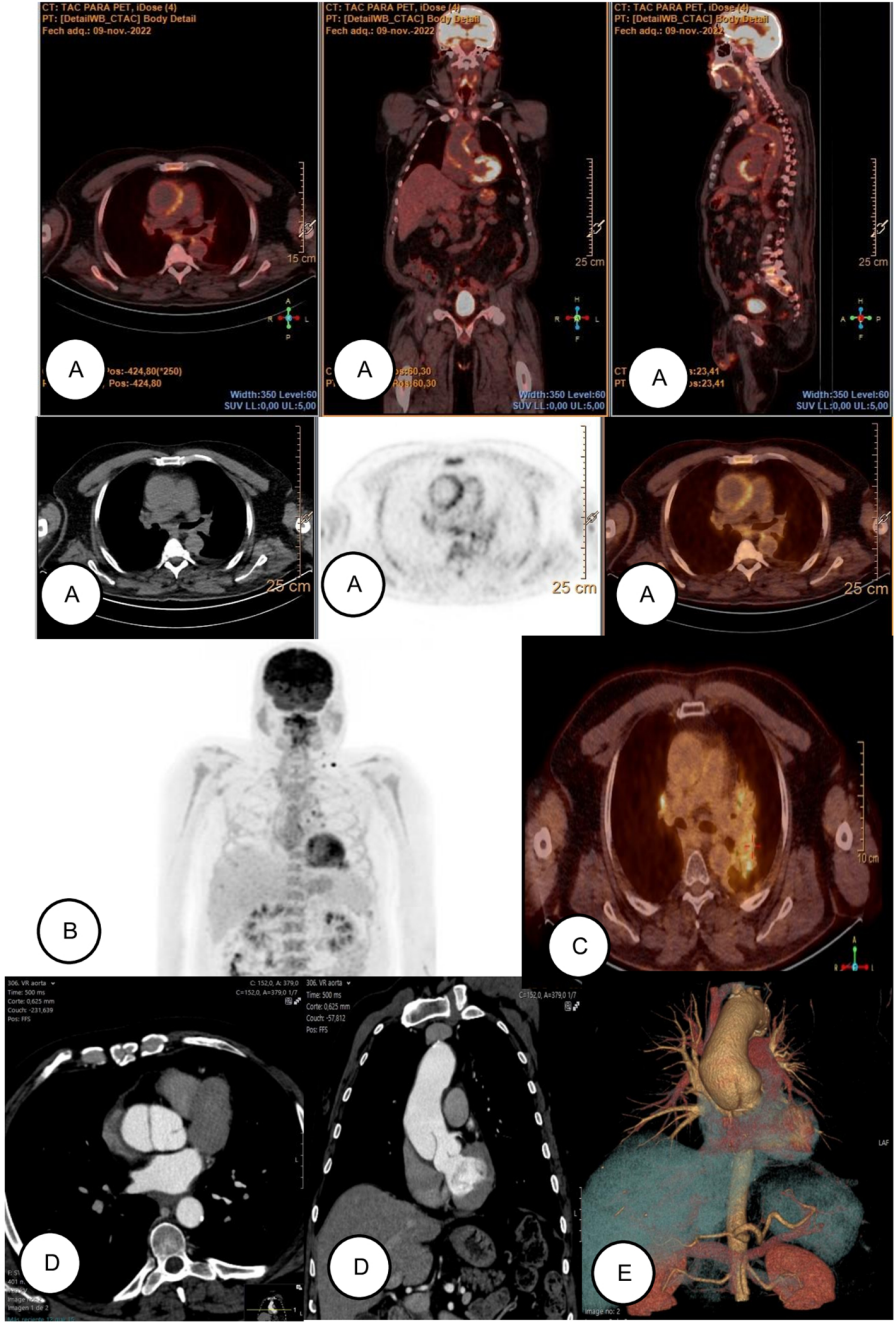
Radiological evolution of vasculitis (images are displayed sequentially from top to bottom and from left to right). (A) November 2022: PET/CT performed at diagnosis, demonstrating high-intensity increased metabolic activity (greater than hepatic uptake) in the thoracic aorta. Imaging was performed using a digital PET/CT scanner (Philips Vereos, Philips Healthcare). Image reconstruction was carried out using a three-dimensional ordered-subset expectation maximization (3D OSEM) algorithm. The administered 18F-FDG dose was 3.5 MBq/kg, corresponding to a total injected activity of 356.3 MBq. Image acquisition started 60 minutes after radiotracer injection. Blood glucose level at the time of injection was 96 mg/dL. Semi-quantitative analysis was performed using standardized uptake values (SUV). SUV was calculated according to the standard formula as the ratio of tissue radioactivity concentration to injected dose normalized by patient body weight. The maximum standardized uptake value (SUVmax) measured at the affected aortic wall was 4.89. The aorta-to-blood pool SUV ratio was 3.0, with a mean blood pool SUV of 1.6. The aorta-to-liver SUV ratio was 1.87, based on a mean hepatic SUV of 2.7. (B) November 2022: coronal maximum intensity projection (MIP) reconstruction demonstrating increased metabolic activity along the aortic wall. (C) May 2023: Follow-up PET/CT with imaging resolution, showing normalization of thoracic aortic metabolism. (D) October 2023: axial and coronal images where intimal flap and aneurysmal dilation are observed in the proximal ascending thoracic aorta. (E) October 2023: Volume rendering 3D reconstruction.
